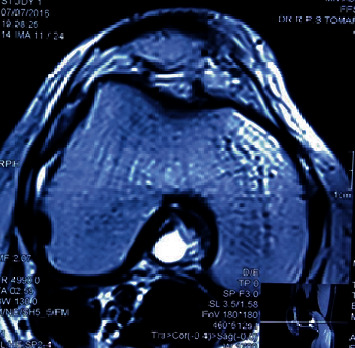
Axial section of MRI showing cyst between the femoral attachment of two cruciate ligaments.
